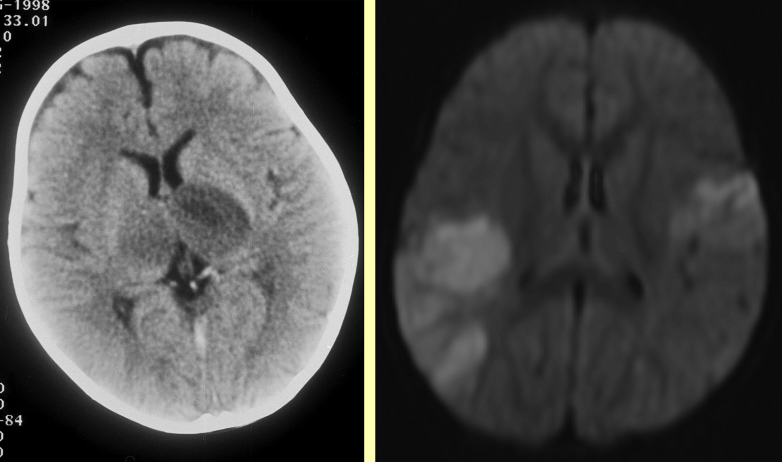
Emergency imaging in bilateral stroke. Left: thalamic infarction in a child with iron deficiency anaemia and venous sinus thrombosis, Right: MRI showing bilateral infarction in a child with acute myeloid leukaemia.
